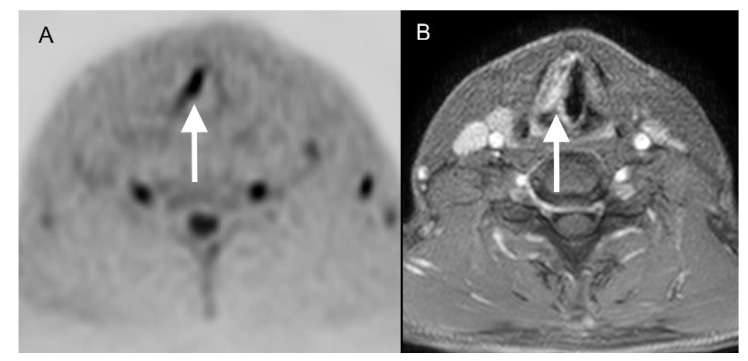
A fifty-year-old patient with a pT1a cN0 cM0 squamous cell carcinoma of the right vocal cord which was detectable for both readers in DWIBS ((A); white arrow) as well as in contrast-enhanced transversal T1-weighted scans with fat saturation ((B); white arrow).
